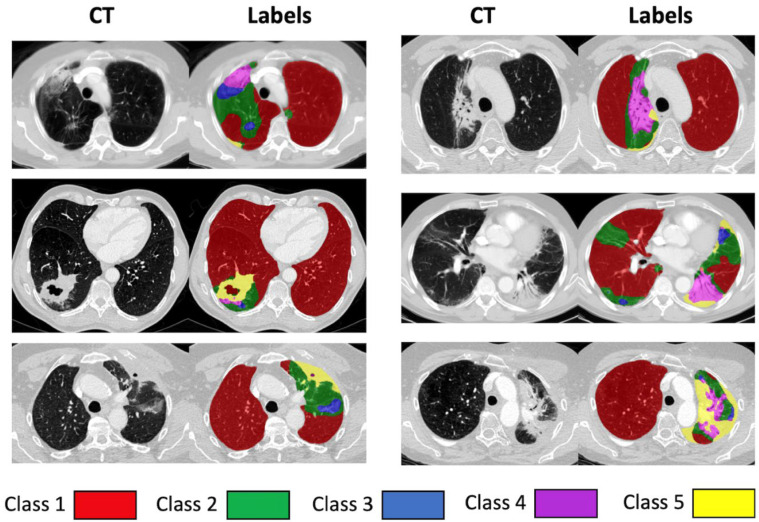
Examples of morphological classes used in parenchymal classification system. Left plain CT. Right automated labels. Class 1; Red, Class 2; Green, Class 3; Blue, Class 4; Magenta; Class 5; Yellow.
